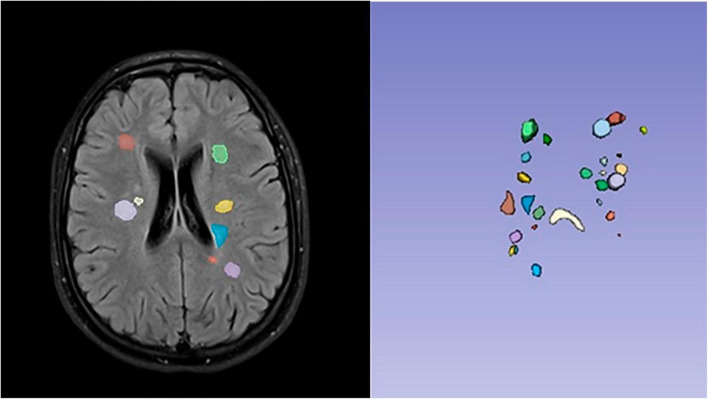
Example patient: segmentation of multiple sclerosis (MS) lesions using 3D Slicer’s semi-automatic tool was guided by T2-FLAIR images. All semi-automated segmentations underwent rigorous validation, including visual inspection by a minimum of three researchers, ensuring accurate and reliable outcomes.
